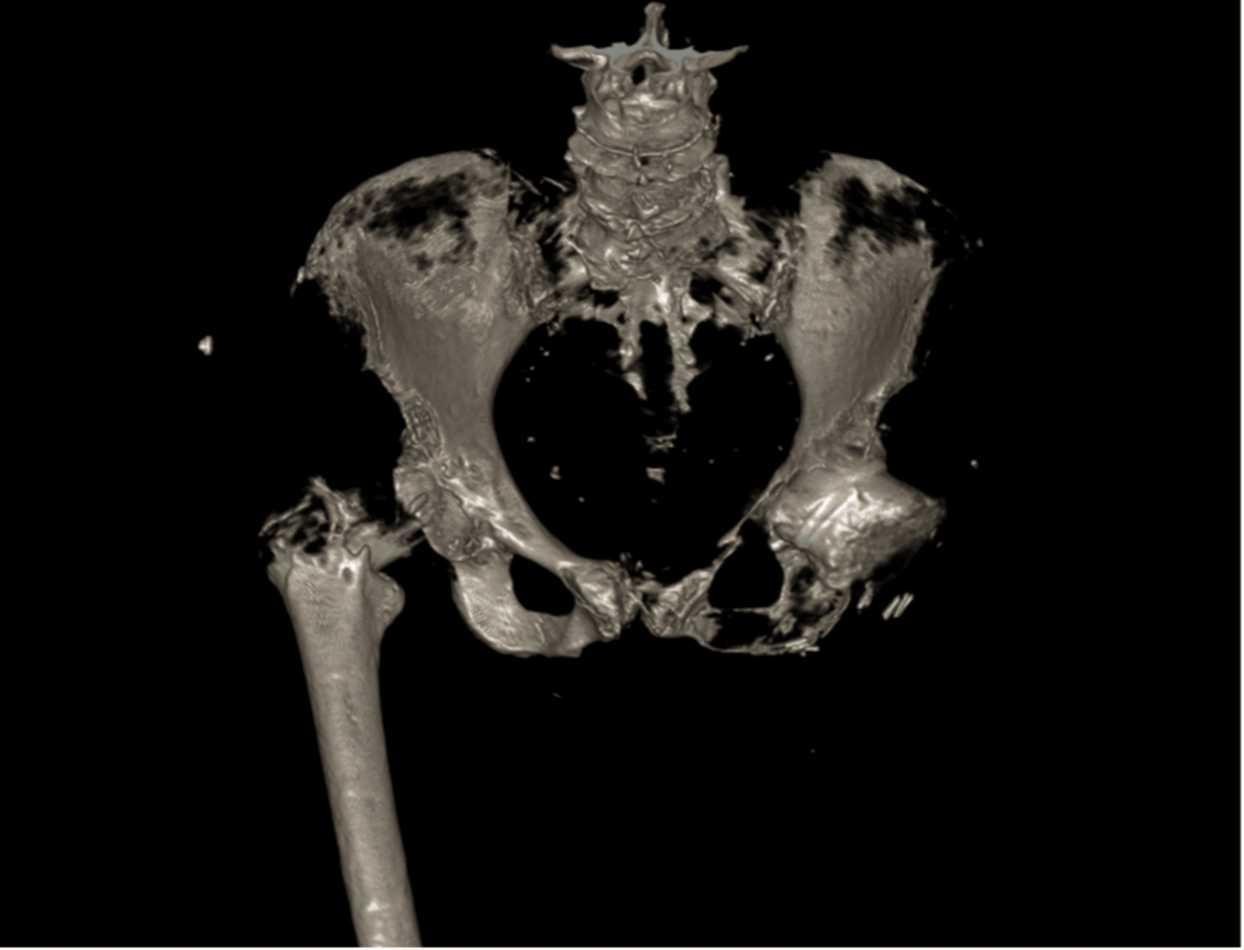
3D CT reconstruction of the right hip fracture
